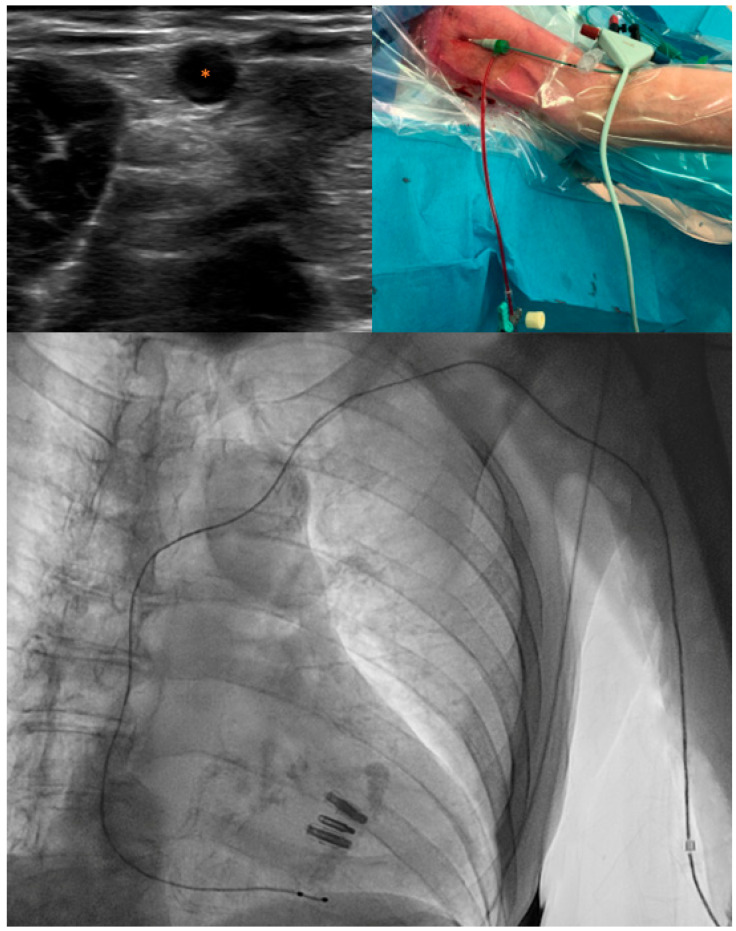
Example of a temporary pacing lead in a left upper arm vein. Upper left: Ultrasound-guided visualization of the vein for temporary pacemaker access. Asterisk denotes the vein. Upper right: 5F temporary pacing lead inserted through a 6F sheath in a left upper arm vein. Lower: Angiographic visualization of the temporary pacing lead trajectory from a left upper arm vein to right ventricular apex.
